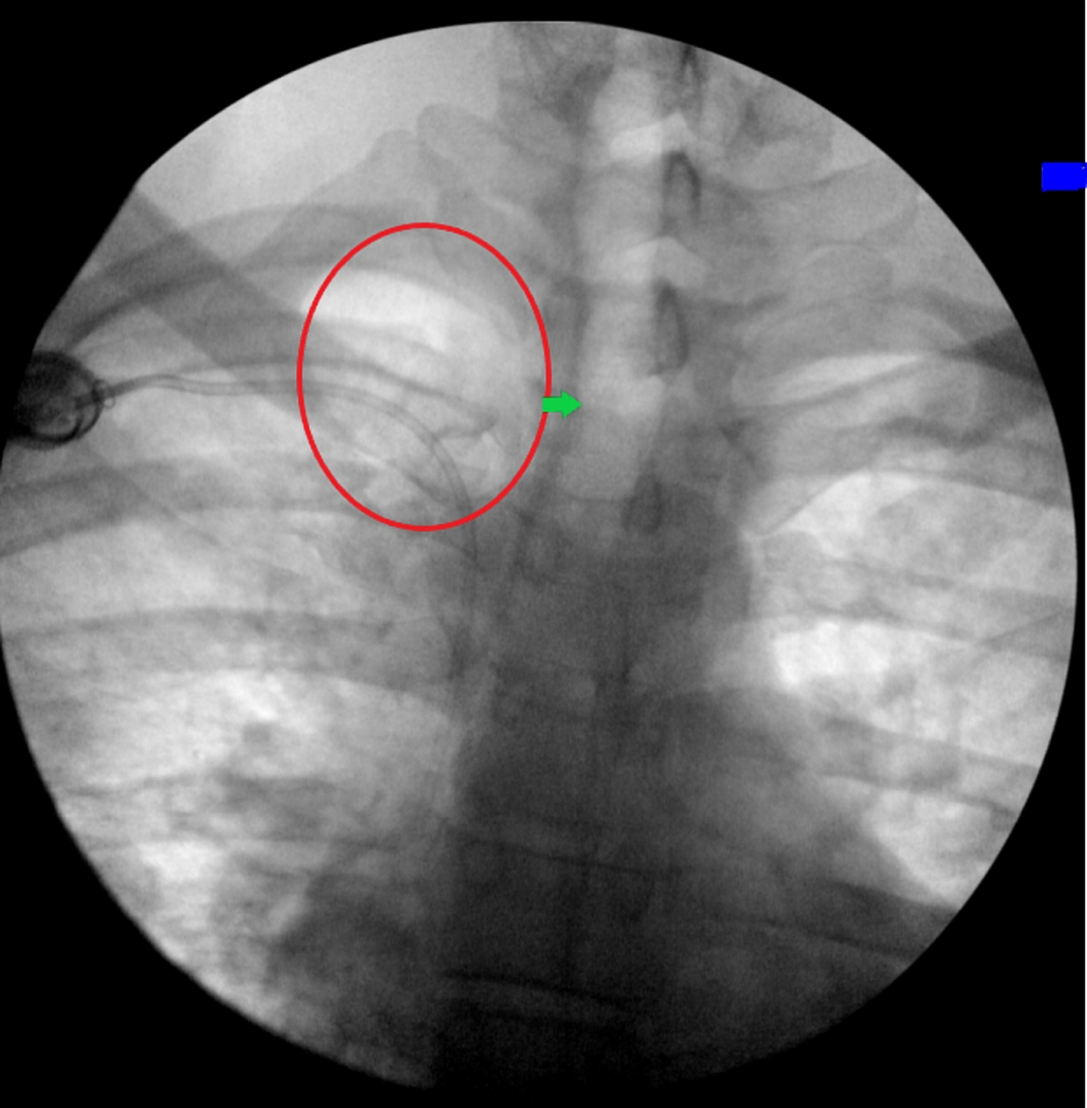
Abdominal computed tomography (CT) scan showing a retroperitoneal mass (red circle) and displacement of the aorta (green arrow)
